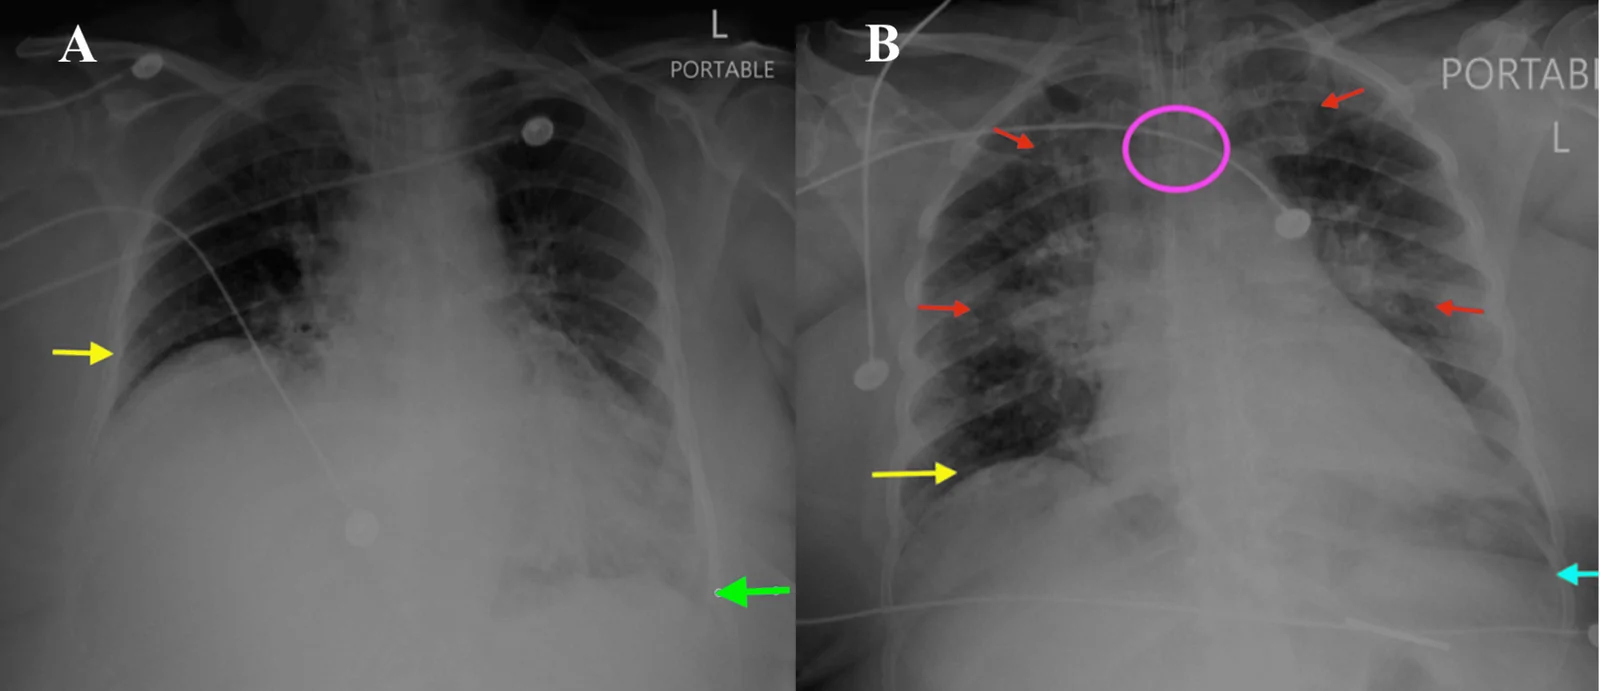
Postoperative chest radiographs Panel A: Postoperative chest radiograph demonstrating acute elevation of the right hemidiaphragm (yellow arrow) with a normally positioned left hemidiaphragm (green arrow), consistent with right phrenic nerve palsy following an interscalene brachial plexus block. The lungs remain clear without focal air-space consolidation, pleural effusion, or pneumothorax. This finding was new compared with the preoperative chest radiograph (Figure 1B) and raised concern for diaphragmatic dysfunction. Panel B: Post-intubation chest radiograph demonstrating bilateral pulmonary edema (red arrows), persistent elevation of the right hemidiaphragm (yellow arrow), a normally positioned left hemidiaphragm (blue arrow), and an appropriately positioned endotracheal tube (purple circle). These findings are consistent with negative-pressure pulmonary edema secondary to acute upper airway obstruction and respiratory failure.
